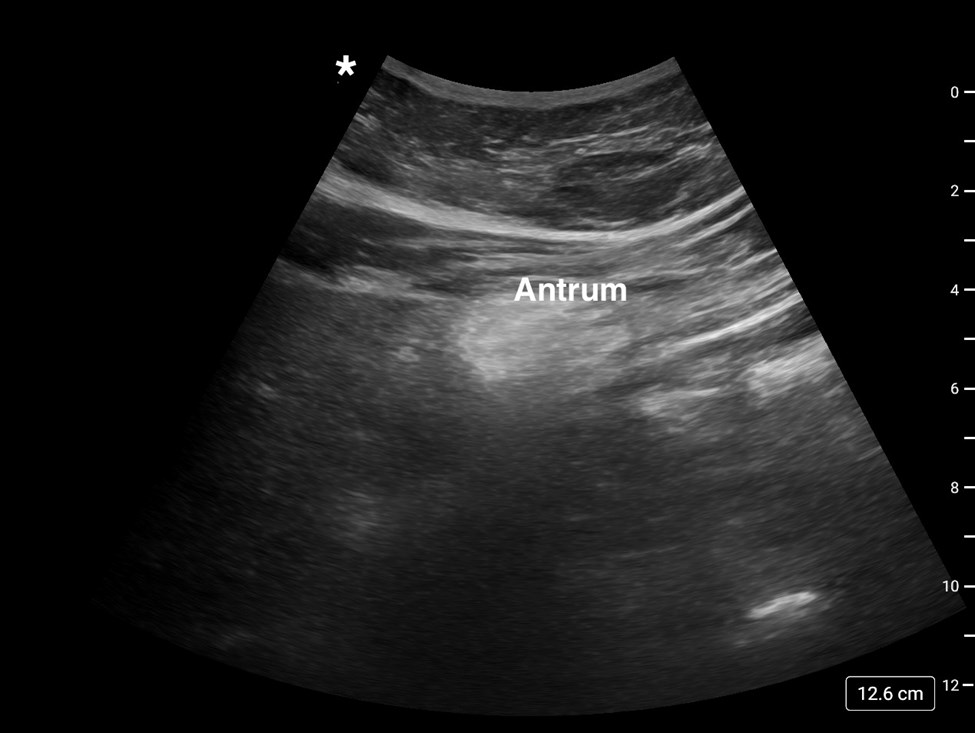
Gastric ultrasound image case #1
